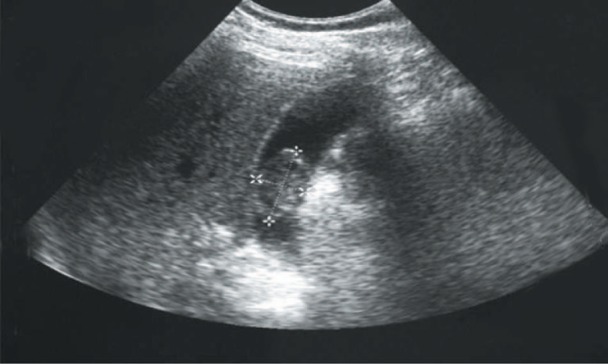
Case 1. Ultrasonography revealing a polypoid intraluminal echogenic nonshadowing gallbladder structure.
